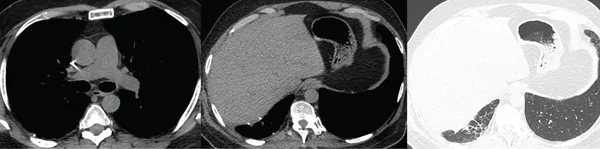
(b) Postoperative chest computed tomography
